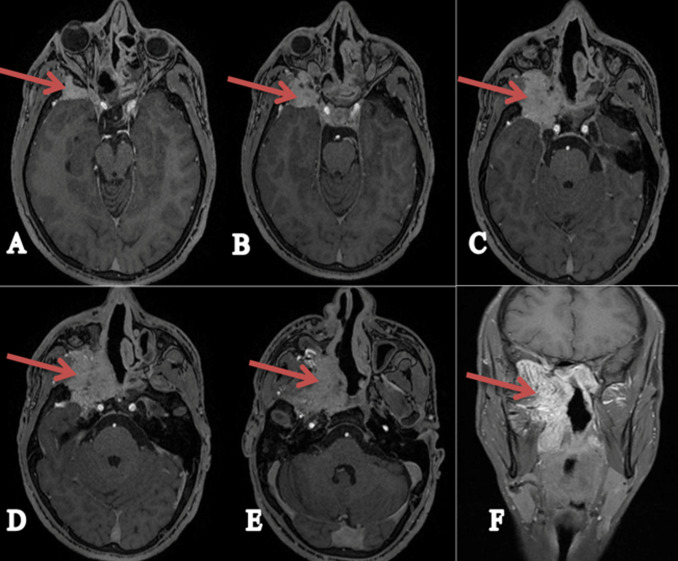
MRI sections in the axial (A+B+C+D+E) + coronal (F) plane sequences T1 with gadolinium injection showing the tumour process on the right side (pterygo-palatine angiofibroma), as shown by the brown arrows
